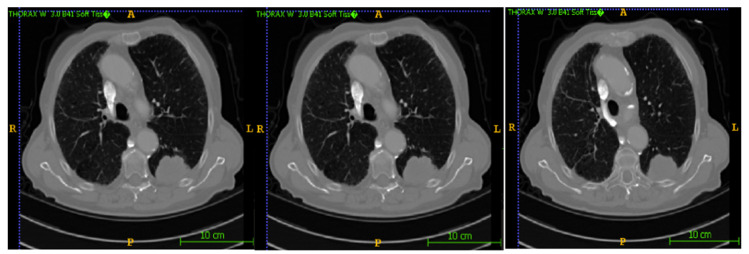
CT slices showing juxta-pleural nodules (Patient QIN_LSC_0064).
